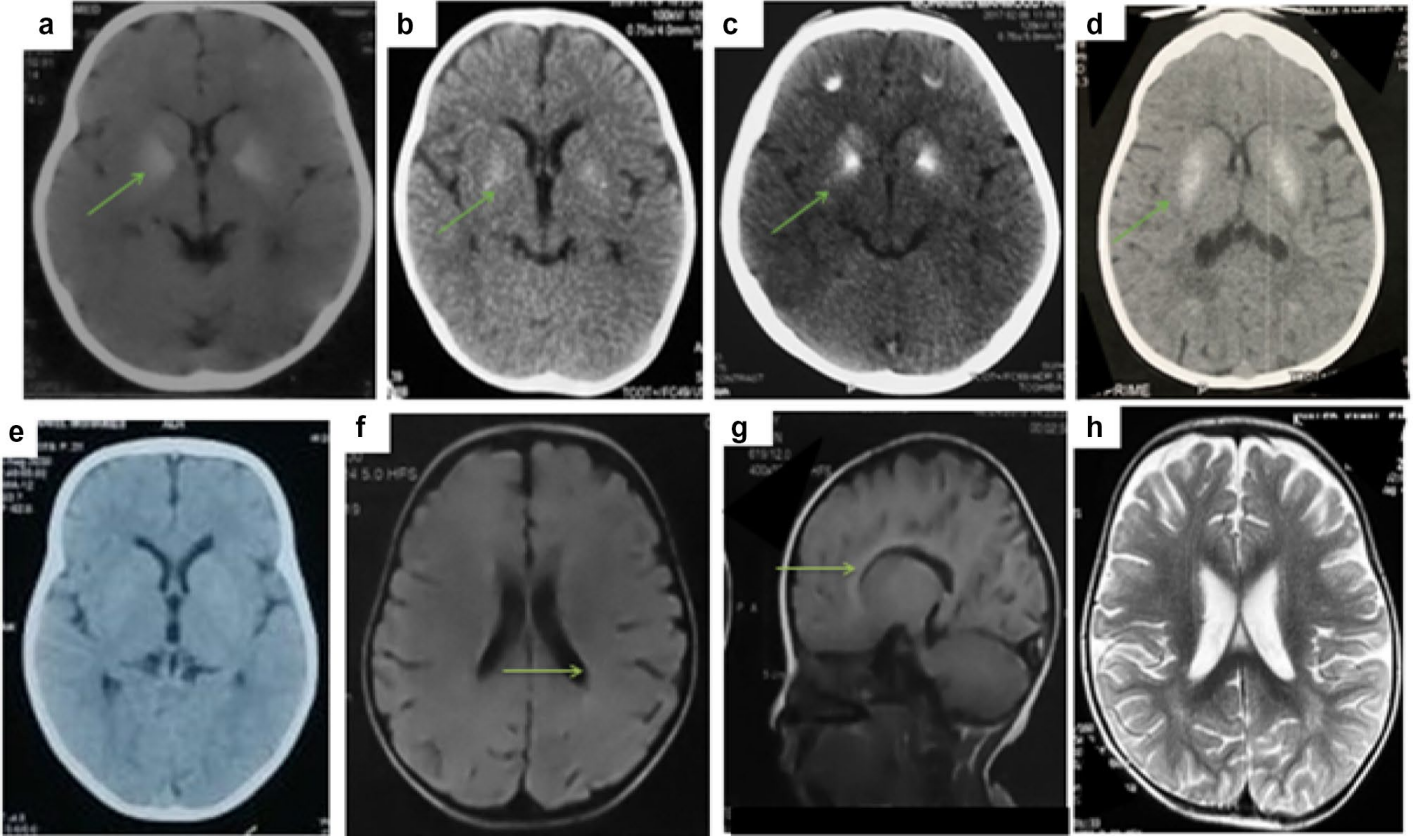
a–d Brain CT scans showing calcification in basal ganglia in the Patients 1, 3, 4 and 5/ respectively. e Brain CT showing absence of calcifications in Patient 2. f, g Brain MRI of Patient 2 showing asymmetry in lateral ventricles and thin corpus callosum. h Brain axial (T2) MRI in Patient 4 showing mild deep white matter signal and minimal cortical and central atrophic changes
